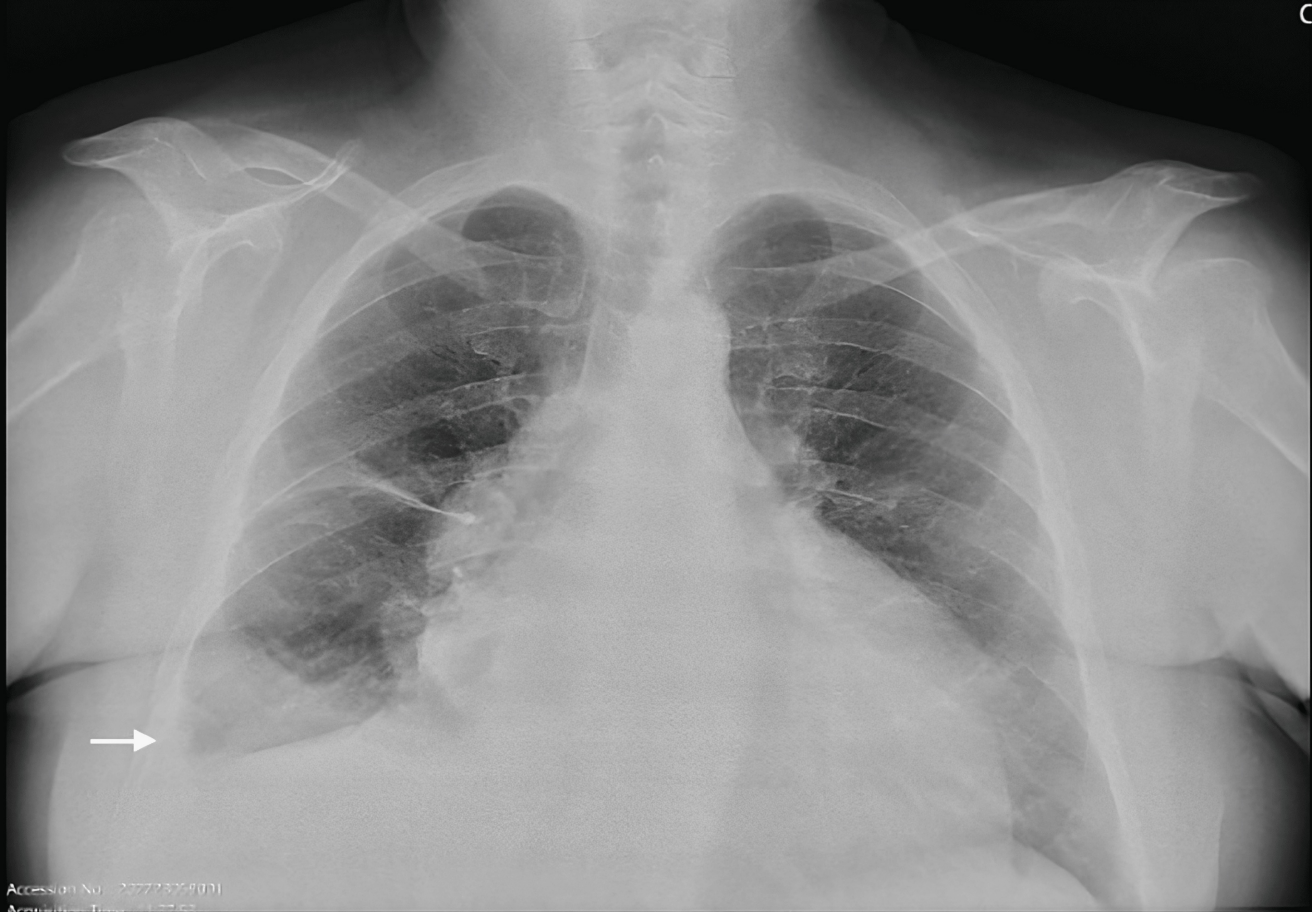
Chest radiography showing a small right-sided pleural effusion with fissural thickening and enlargement of the cardiac silhouette.
